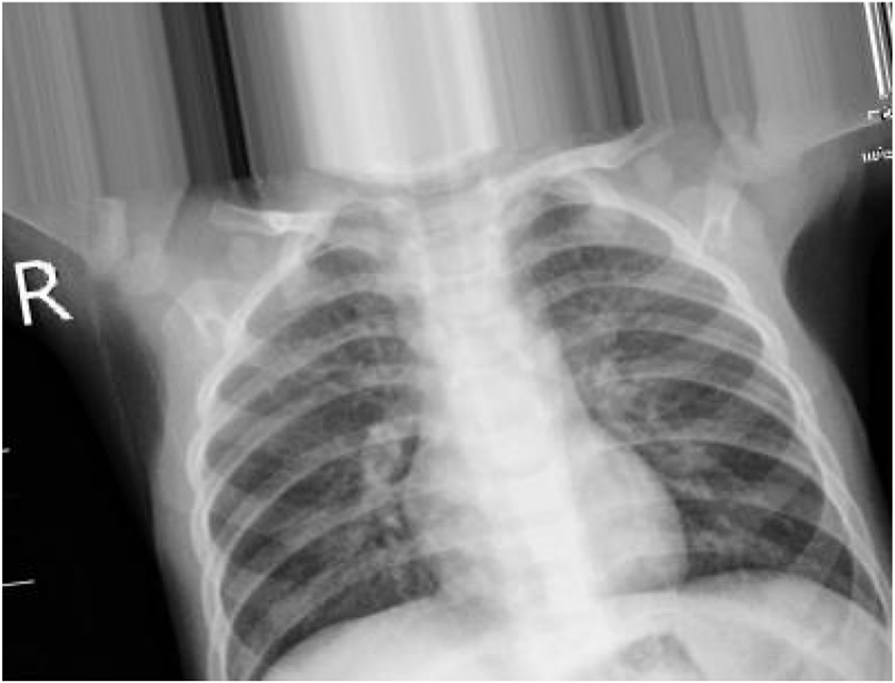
Sample of chest X-ray image of a BP patient
